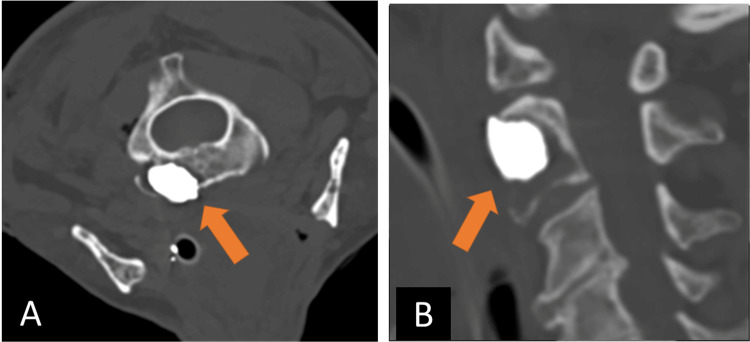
Intraoperative images during C2 lesion biopsy and kyphoplasty. Near complete filling of the lesion cavity with PMMA cement. (A) axial bone window CT; (B) sagittal bone window CT. PMMA: Polymethyl Methacrylate
